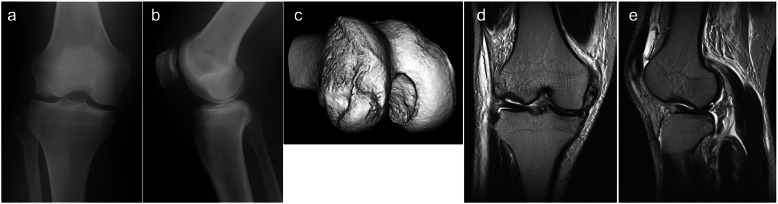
X-ray, computed tomography scan and magnetic resonance imaging before surgery. a Anteroposterior, b Lateral radiographs, c A 3-dimensional computed tomography of the right knee demonstrating a large depression fracture of the lateral femoral condyle. d Coronal T2-weighted, e Sagittal T2-weighted magnetic resonance imaging of the right knee taken before surgery demonstrated a large osteochondral defect of the lateral femoral condyle and superficial medial collateral ligament rupture
